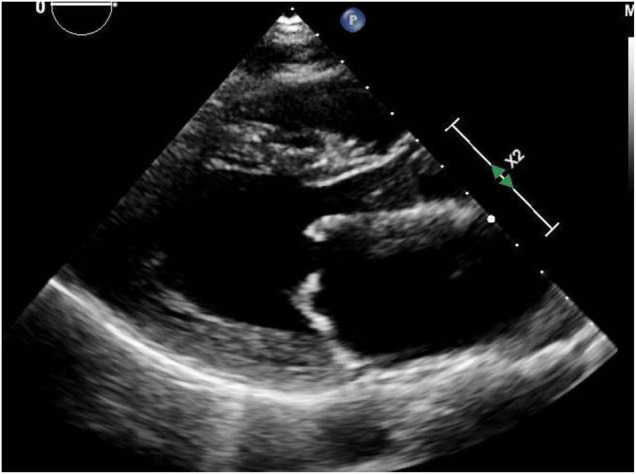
Mitral stenosis: Representative parasternal long axis view during showing mitral stenosis in a woman with rheumatic heart disease. Both leaflets are affected with thickening and also restriction of their movement.
